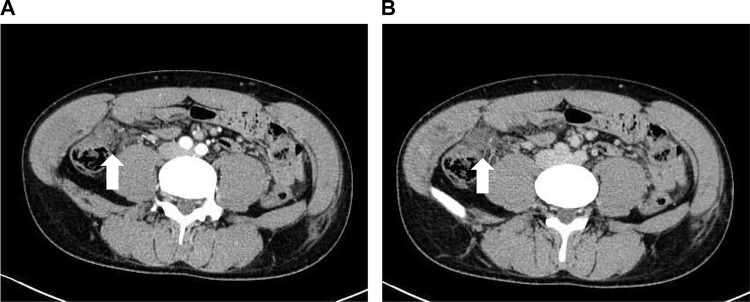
Mesenteric hematoma. Abdominal arterial (A) and portal (B) phase cross-sectional contrast-enhanced CT show a hematoma on the distal side of the ileocolic artery (arrows).
